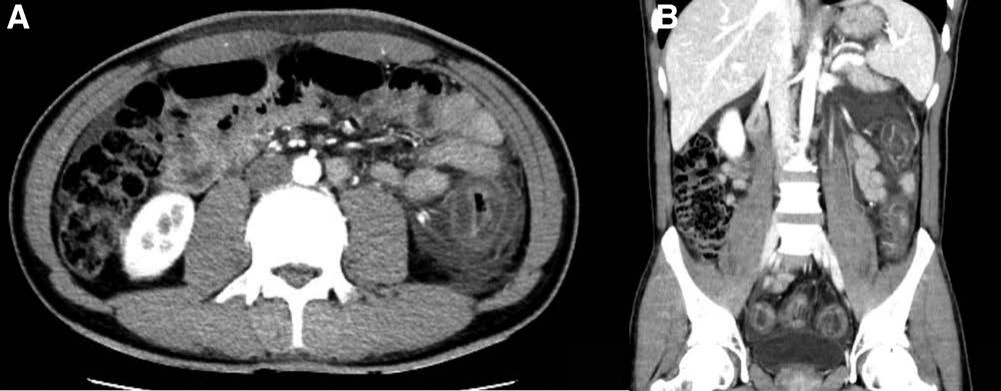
Oedema and thickening of the rectum and colon walls were observed on mesenteric computed tomography angiography at admission.
